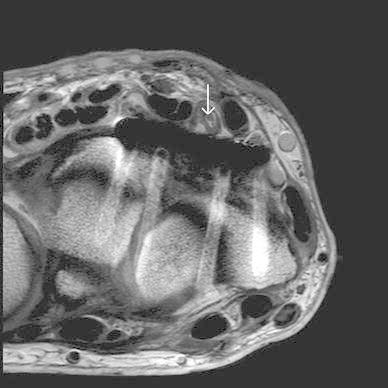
MRI showing FPL rupture (*white arrow*) and close proximity of the flexor tendon to the volar plate (MRI performed before FDP to index finger rupture)
